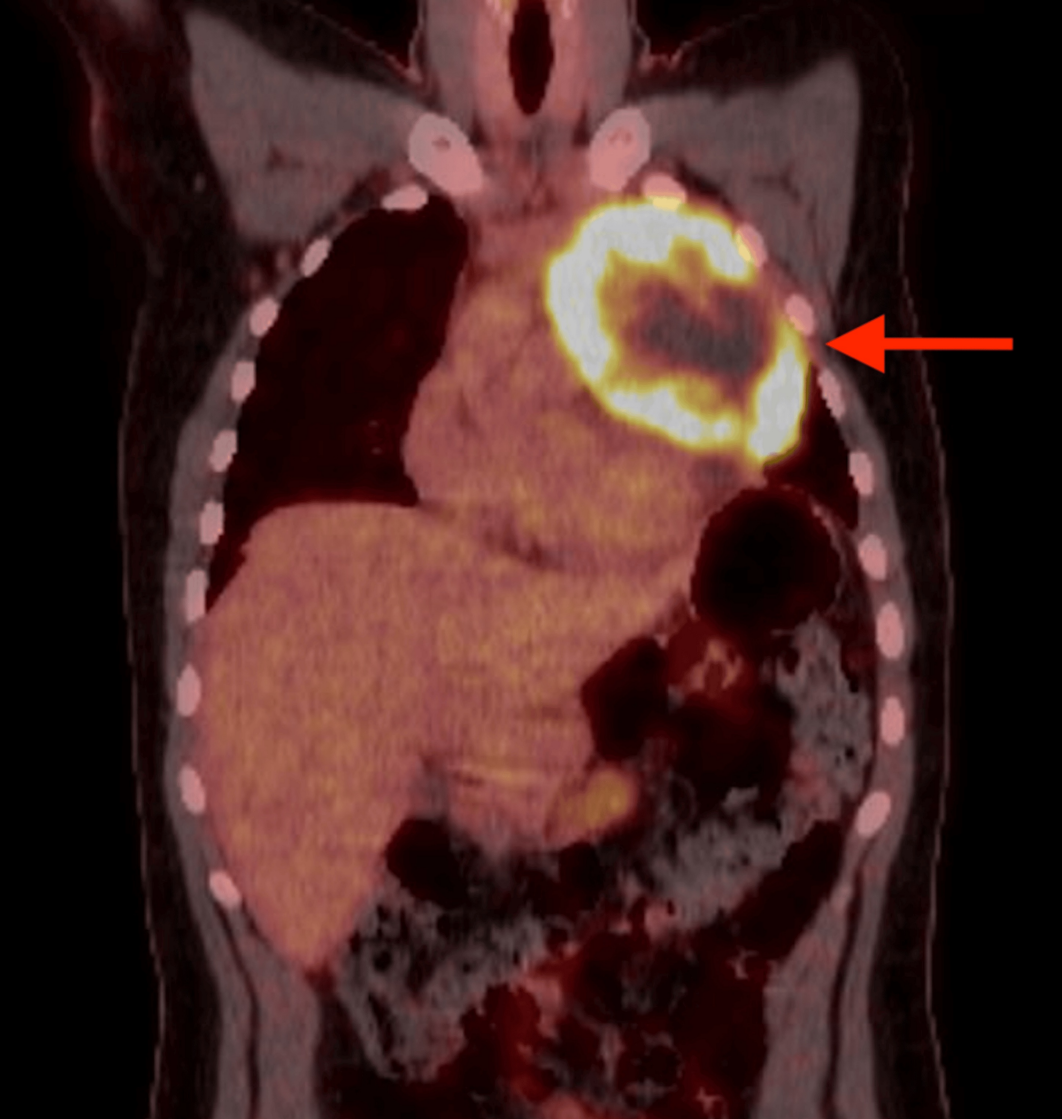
FDG PET-CT scan showing a left-sided anterior mediastinal mass with increased FDG uptake (arrow). FDG PET-CT: Fluodeoxyglucose Positron Emission Tomography-Computed Tomography
